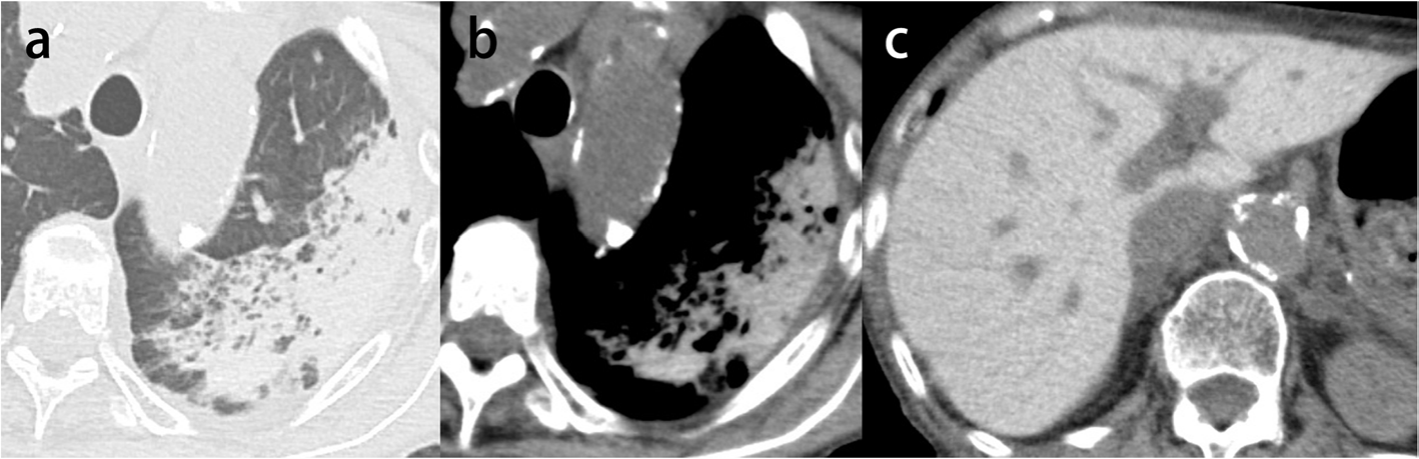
Amiodarone pulmonary toxicity in an 85-year-old woman. a CT image shows an extensive area of consolidation in the left upper lobe. b Mediastinal window image shows consolidation with high attenuation. c The liver shows increased attenuation, which is compatible with amiodarone deposition
